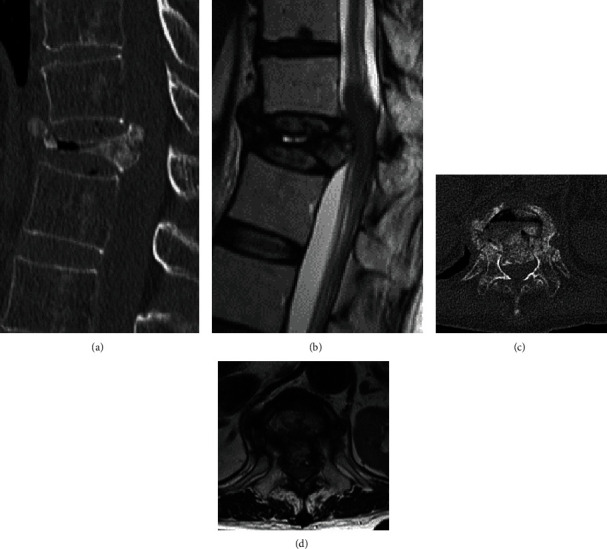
Preoperative thoracolumbar computed tomography (CT) images and magnetic resonance images (MRIs) of a 66-year-old woman with collapse of the 12^th^ thoracic (T12) vertebra. (a) sagittal CT image; (b) sagittal T2-weighted MRI; (c) axial CT image at the T12 vertebra; (d) Axial MRI image at the T12 vertebra.
